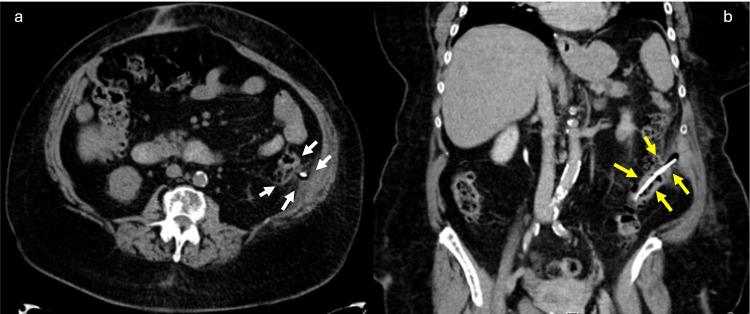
(a) Abdominal axial CT scan showing a tubular structure in the lumen of the sigmoid colon, extending to the peritoneal membrane on the left abdominal wall (white arrows). (b) Coronal section showing a plastic biliary stent, extending to the peritoneal membrane on the left abdominal wall (yellow arrows).
